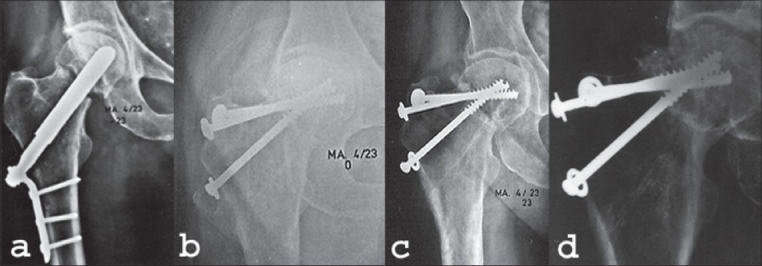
Refixation of a delayed union of the femoral neck in a 57-year-old woman. (a) Two months after Böhler nail shows distraction of the fracture. (b) postoperative X-ray after refixation with cancellous lag screws, acting as a tension band. (c) Five months after refixation. Fracture has healed. (d) followup at 4.5 years after refixation shows avascular necrosis of the head of the femur. The patient did not need a total hip arthroplasty until 14 years after refixation
